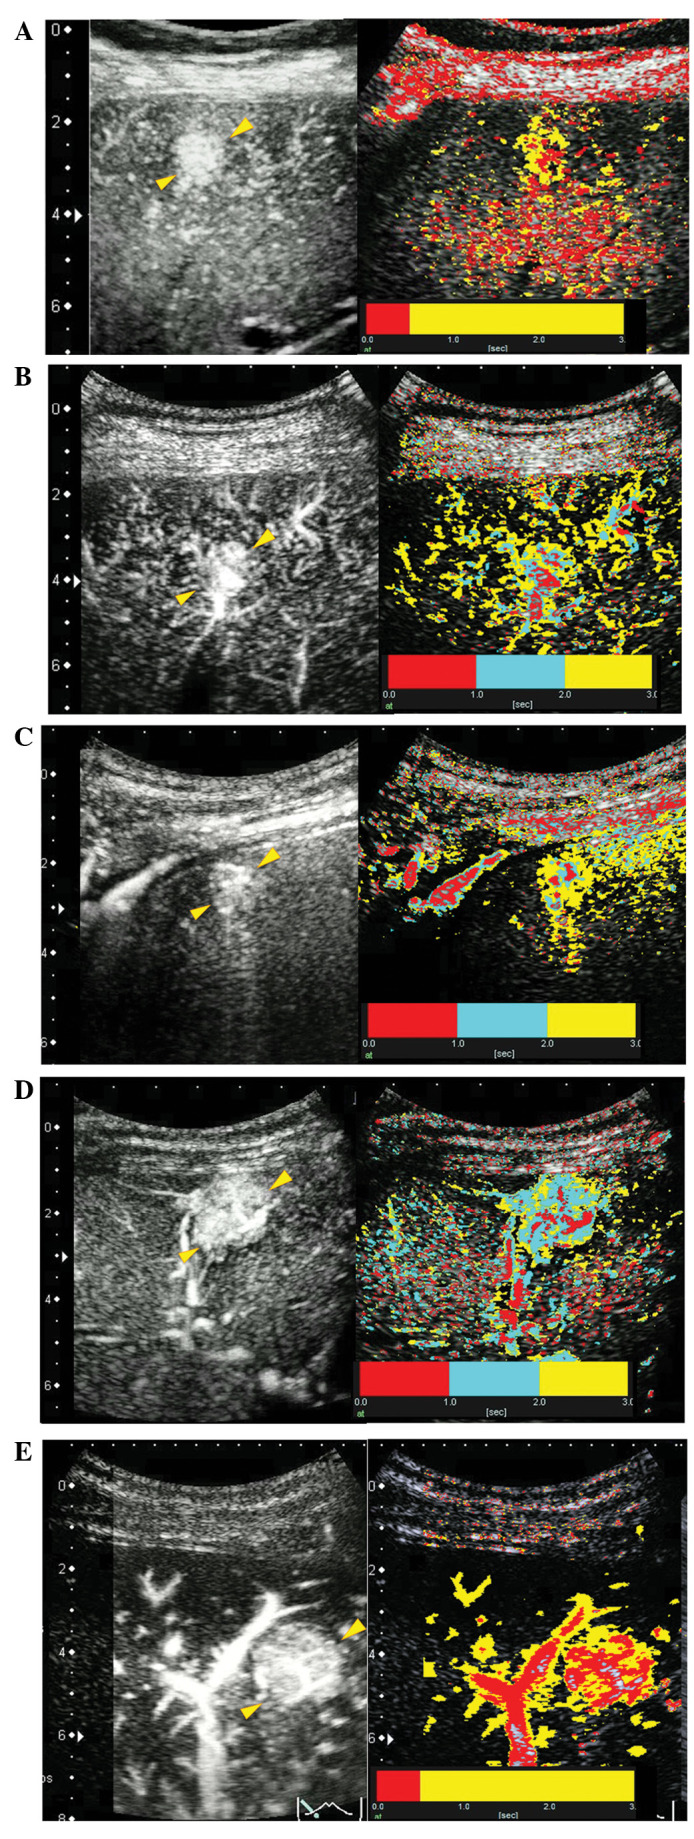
Ultrasound (US) imaging of focal nodular hyperplasia (FNH) in 5 cases. Left, micro-flow imaging (MFI) and right, arrival time parametric imaging (At-PI). In (A) case 1 and (B) case 2, a spoke-wheel pattern was visible in At-PI but not in MFI. In (C-E) cases 3, 4 and 5, respectively, a spoke-wheel pattern was visible in MFI and At-PI. Arrowheads indicate the tumor.
